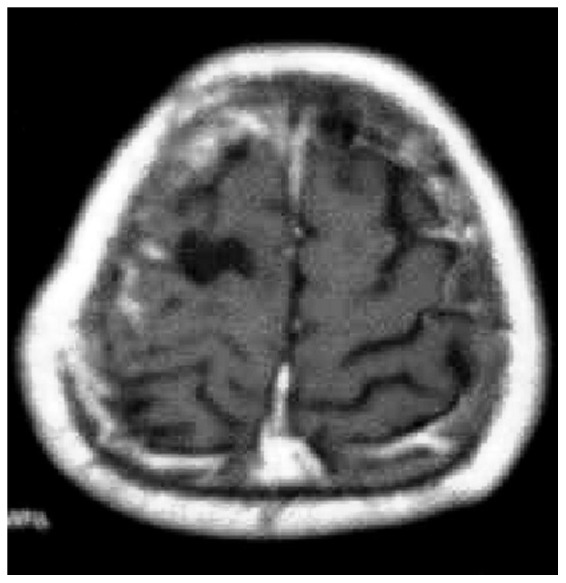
Post-operative magnetic resonance imaging (MRI) of a parasagittal meningioma in the central gyrus region.
